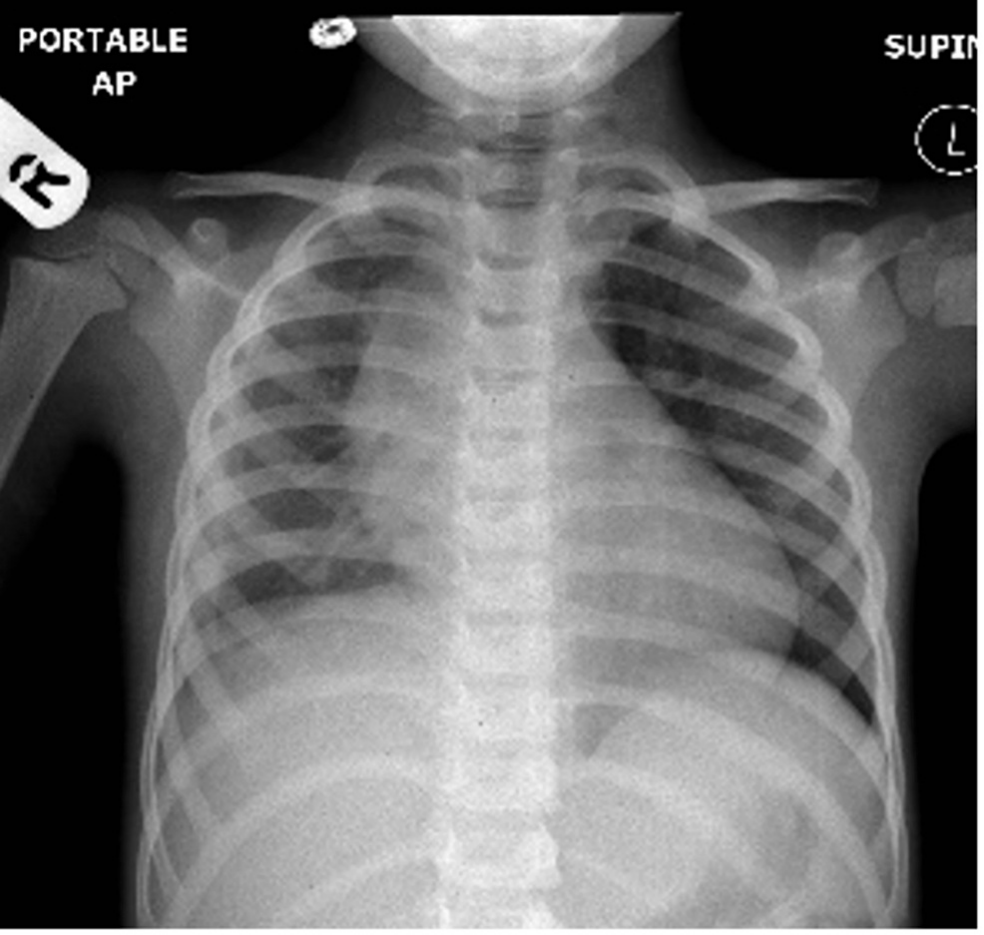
Plain chest X-ray, anteroposterior view, showing a marked resolution of the effusion, thick pleural surface, reduced lung volume on the right side, and potential widened mediastinum.
